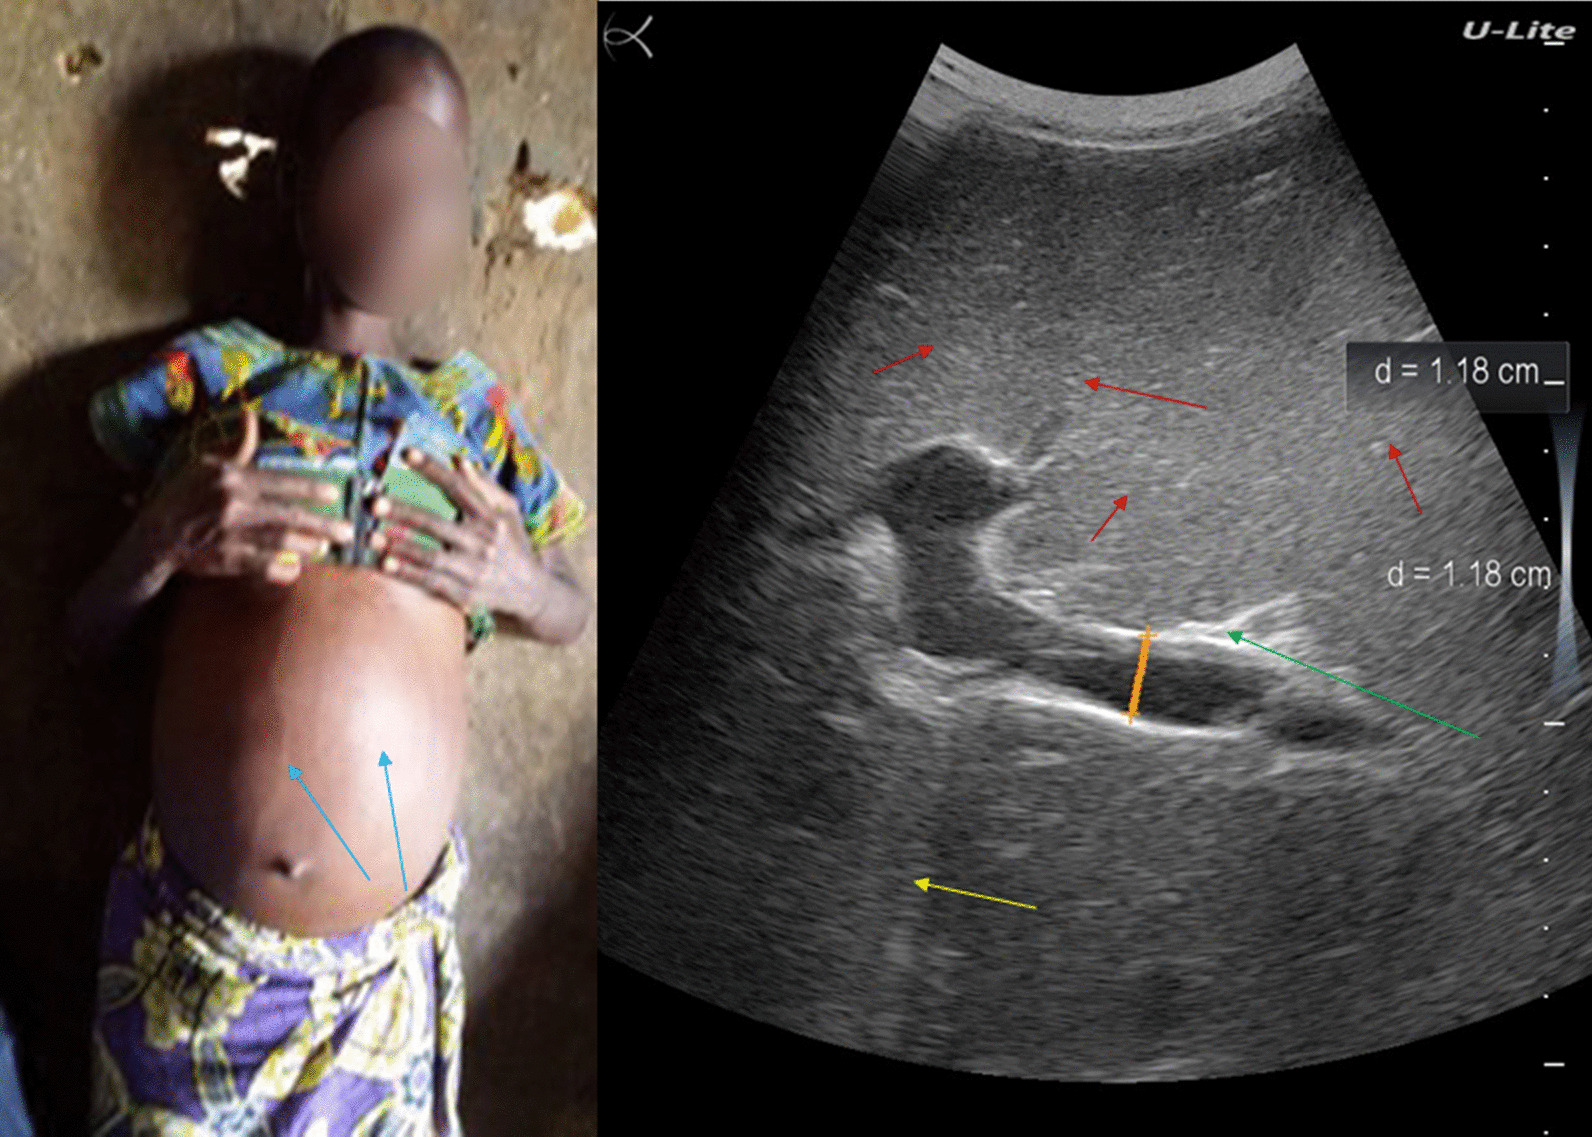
Patient #5 (left) showing collateral veins and large splenomegaly (blue arrows). Ultrasound examination (right) showing starry night sky liver pattern, liver architecture and heterogeneous echotexture of liver parenchyma (red arrow), decreased definition of portal vein wall (green arrow), and posterior reinforcement (yellow arrow)
